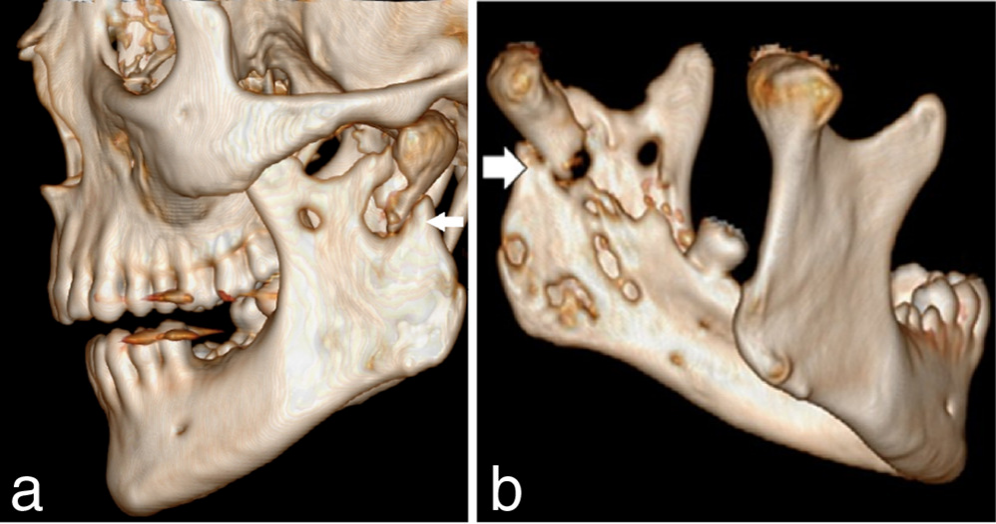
Preoperative 3D-CT volume-rendered reconstructed images demonstrating osteomyelitis and a pathological fracture of the mandibular condyle, which is marked with arrows.
